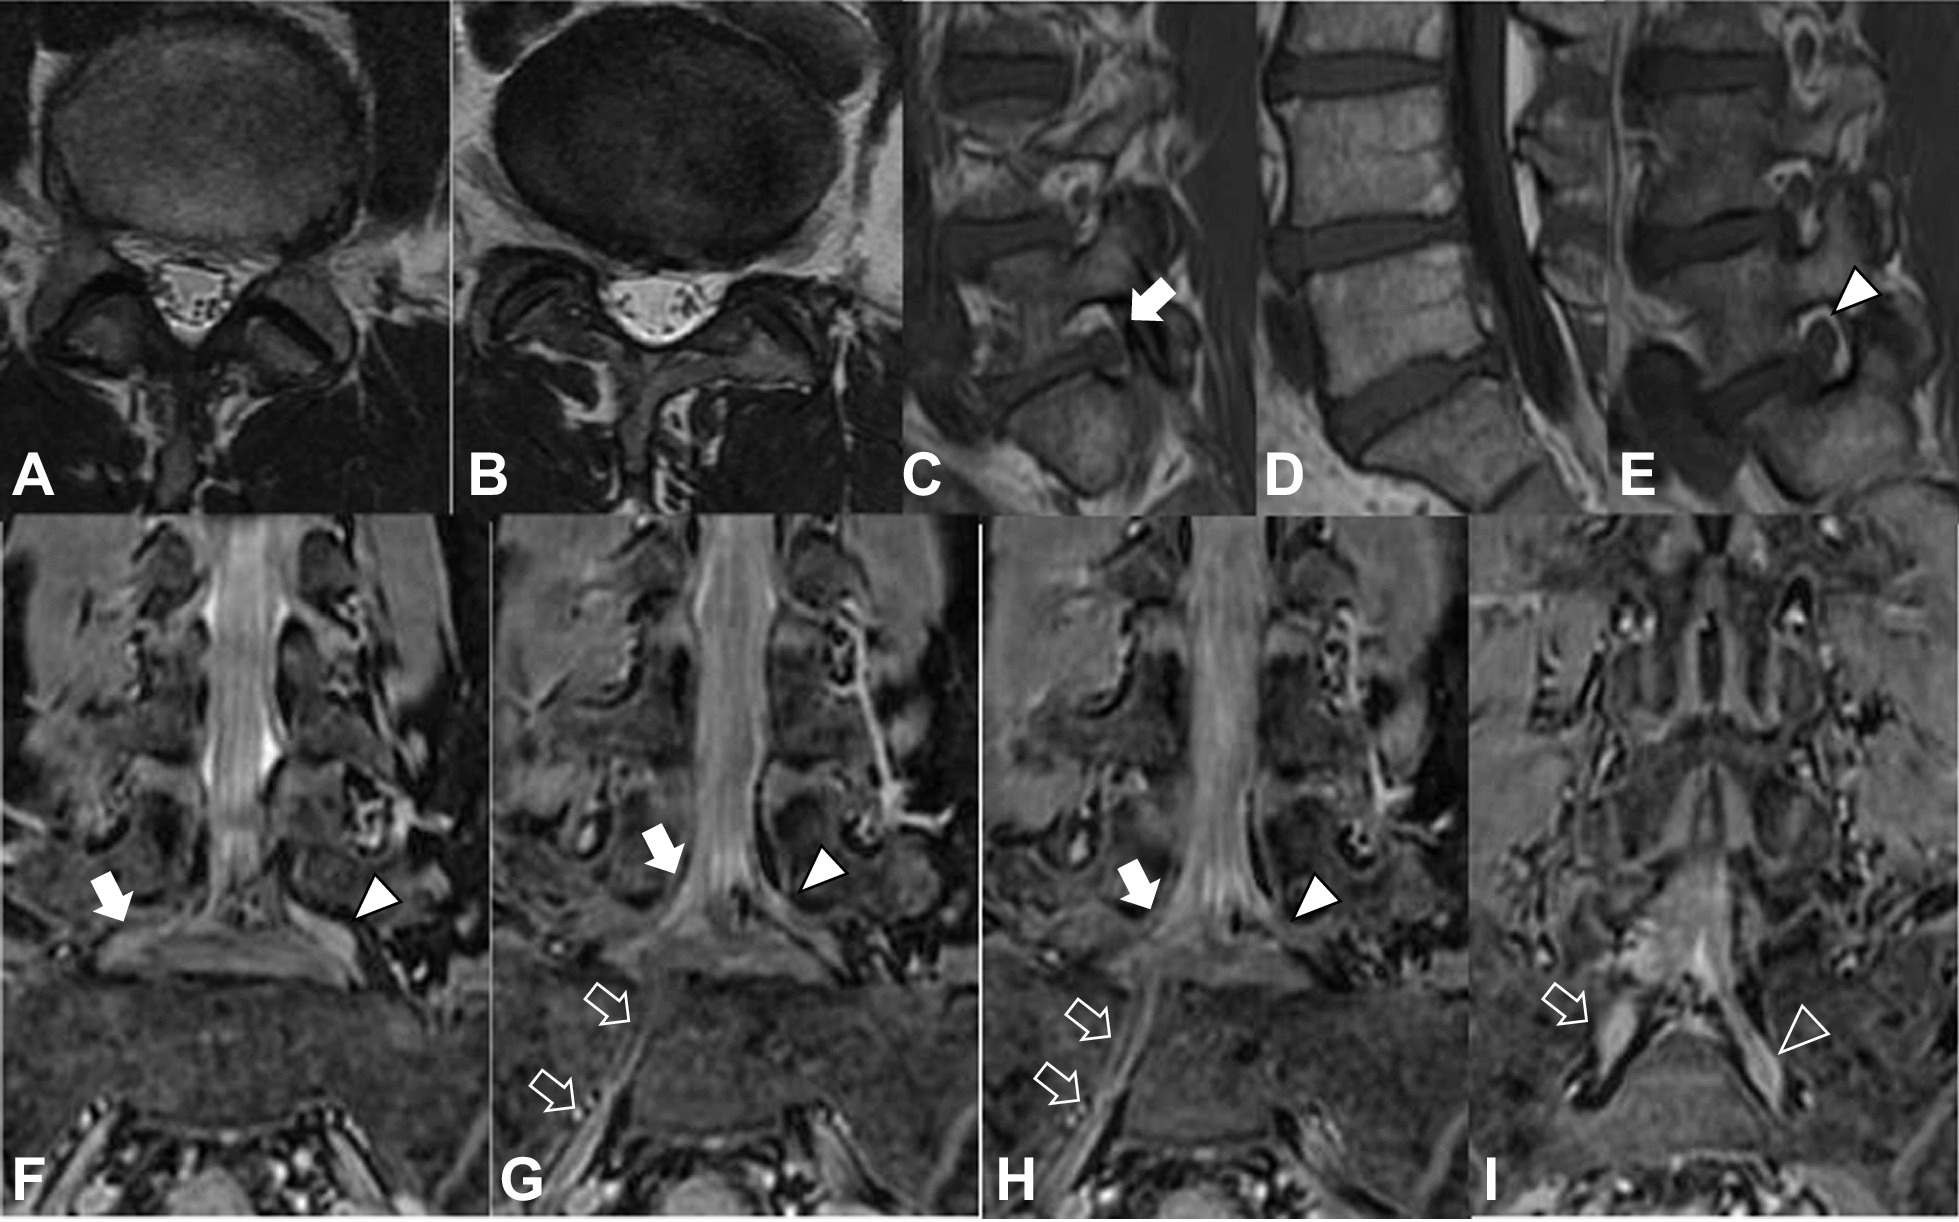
Magnetic resonance imaging findings showing no disc herniation on T2-weighted imaging (a L4–5 axial; b L5–S axial), no foraminal stenosis on T1-weighted imaging (c right sagittal; d central sagittal; e left sagittal), and a right conjoined lumbosacral nerve root (white solid arrow and white outline arrow) on T2-weighted imaging (f–i coronal). The right L5 nerve root (white solid arrow) is located significantly lower in the intervertebral foramen than the left L5 nerve root (white solid triangle) and joins a common trunk with the S1 nerve root (white outline arrow). The right S1 nerve root branches more proximally than the left S1 nerve root (white outline triangle). *MRI* magnetic resonance imaging
